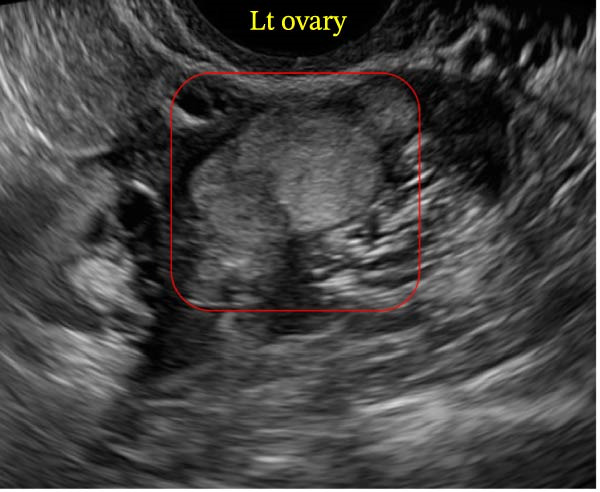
Transvaginal ultrasound showing a hyperechoic lesion in the left ovary. The appearance is consistent with either a hemorrhagic cyst or echogenic thyroid‐like tissue suggestive of struma ovarii. No significant free fluid was observed around the ovaries or in the pouch of Douglas during this examination.
